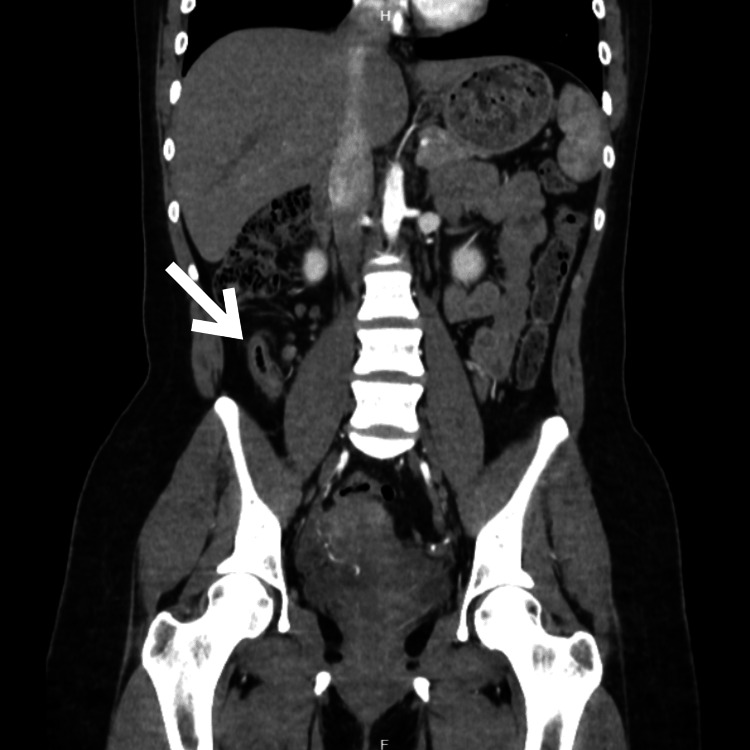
Computed tomography scan showing a dilated appendix, coronal view (white arrow).
